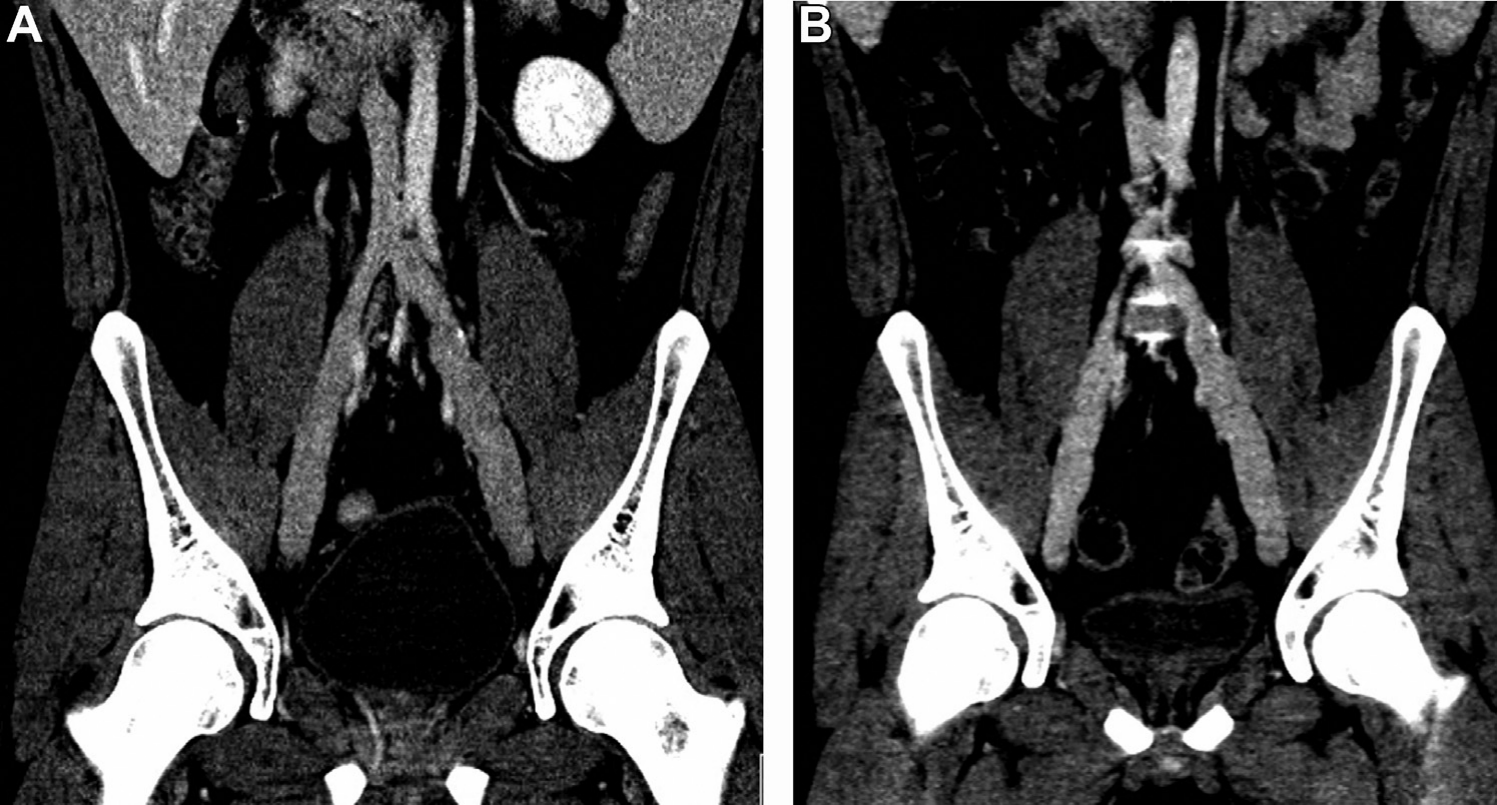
Postoperative images. A, Coronal computed tomography image showing bilateral external iliac veins 6 months after repair. B, Coronal computed tomography images showing bilateral external iliac veins 4 years after repair.
